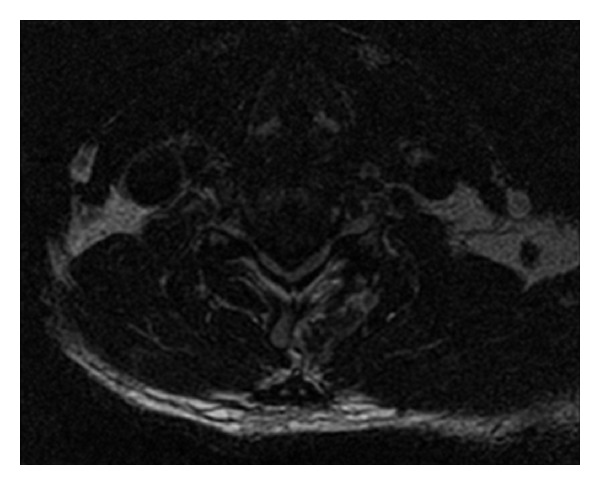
Preoperative axial T2-weighted MRI showing severe C5-6 cord compression by a massive disc herniation.
